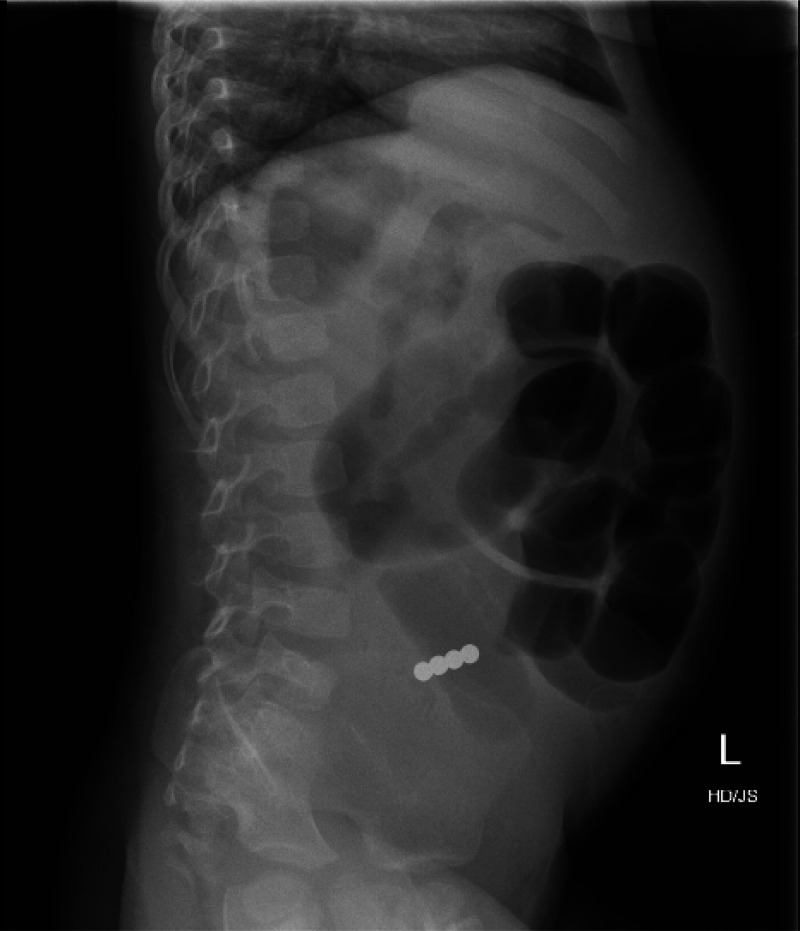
X-ray abdomen (lateral view), multiple rare earth magnets
